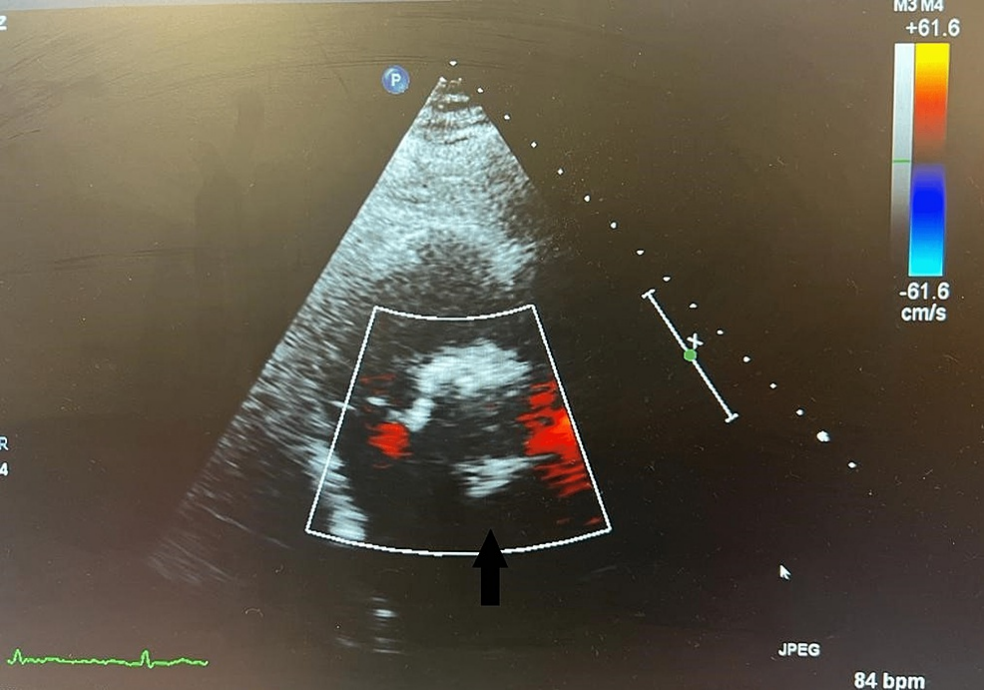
Echocardiography Arrow indicates implanted aortic valve
